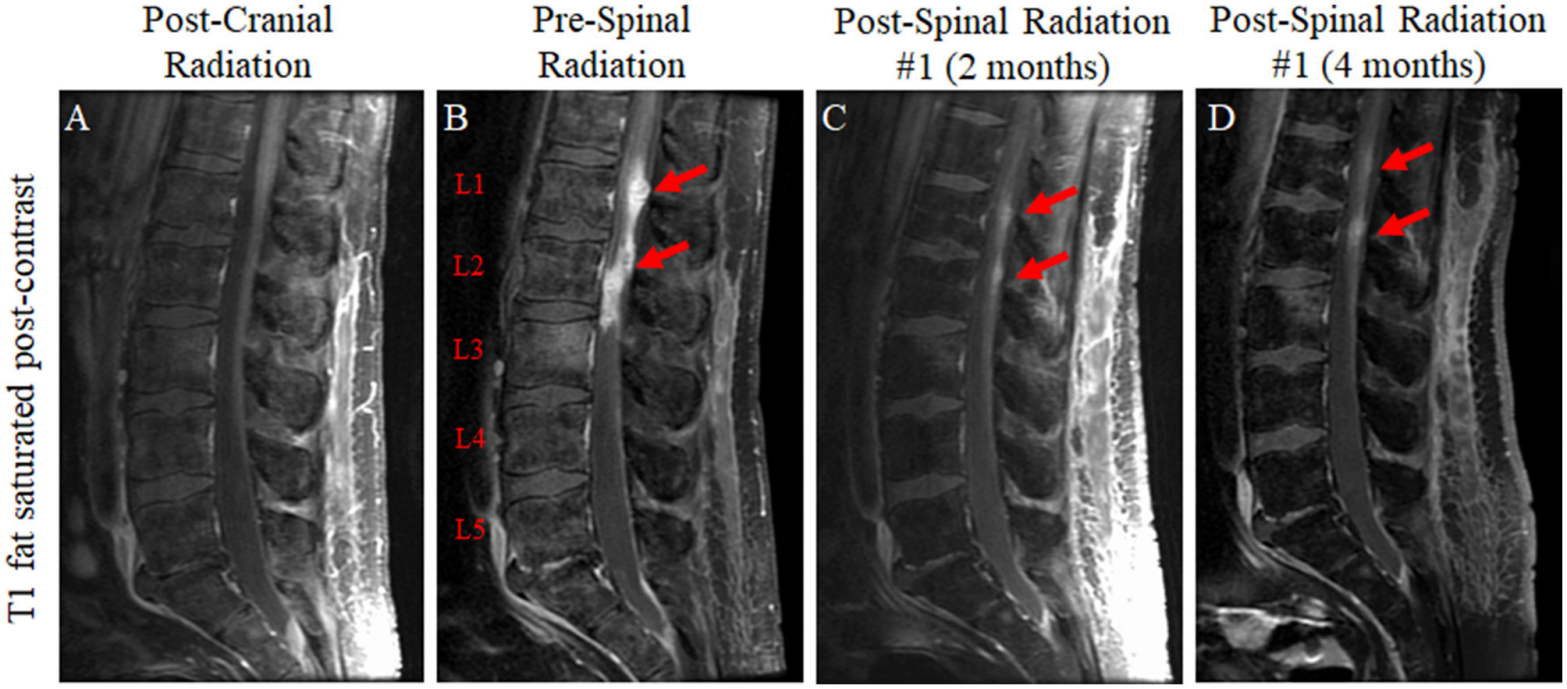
T1-weighted fat-saturated post-contrast sequences of the lumbar spine for the patient in case 2 immediately following radiation to the brain lesion (A); 7 months later following the development of new lower back pain which revealed leptomeningeal drop metastases at the level of T12 extending to the terminus of the thecal sac (T12 to L3 predominant lesions represented graphically) (B); approximately 2 months after the completion of 30 Gy of radiation in 10 fractions to T10-S4 with a significant reduction in lesion size (C); and approximately 4 months after the completion of spinal radiation (D).
